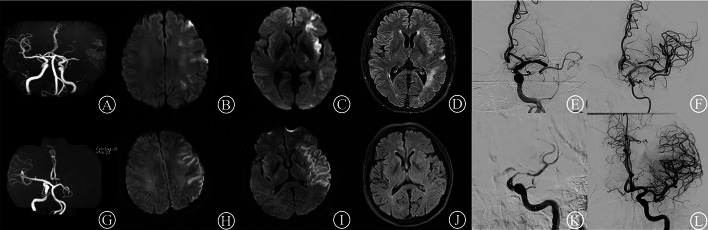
Representative images of different patients with ACALVOS. A-F: A patient with M1 segment occlusion of the left middle cerebral artery and DWI-FLAIR mismatch. The preoperative DWI-ASPECTS was 3 points, and the post-operative mTICI grade was 2b following EVT; Figure G-L: A patient with left internal carotid artery intracranial segment occlusion and DWI-FLAIR mismatch. The preoperative DWI-ASPECTS was 4 points, and the post-operative mTICI grade was 3 following EVT
